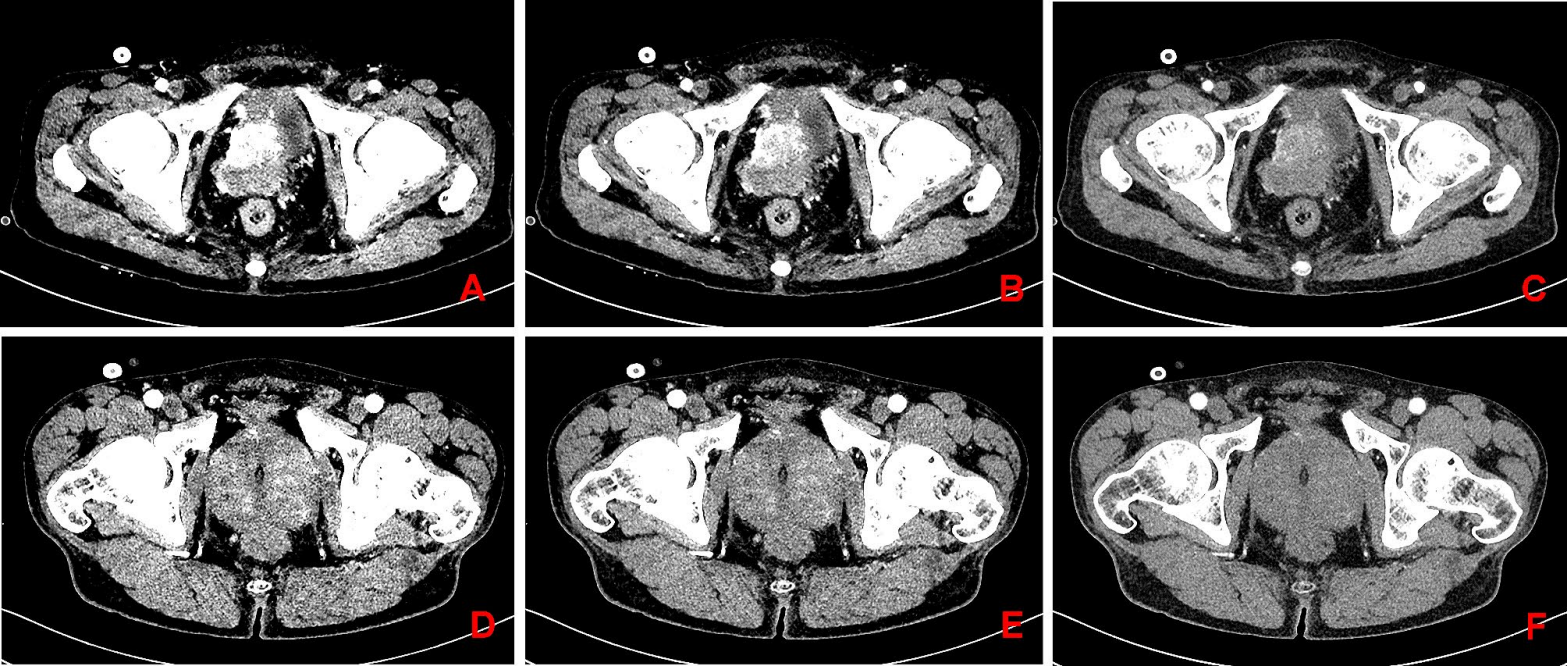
Comparison of CT values and CNR at 40 keV, 50 keV, and conventional linear blending images in patients with prostate cancer and prostate hyperplasia (Window Width: 300 / Window Level: 40). (A-C) The CT values of prostate cancer at 40 keV (A), 50 keV (B), and conventional linear blending image (C) were 246.8 HU, 175 HU, and 84.6 HU, respectively; CNRs were 6.75, 5.90, and 2.55, respectively. (D-F) The CT values of prostate hyperplasia at 40 keV (D), 50 keV (E), and conventional linear blending image (F) were 101.5 HU, 81.9 HU, and 54.5 HU; CNRs were 1.12, 0.75, and 0.11, respectively
